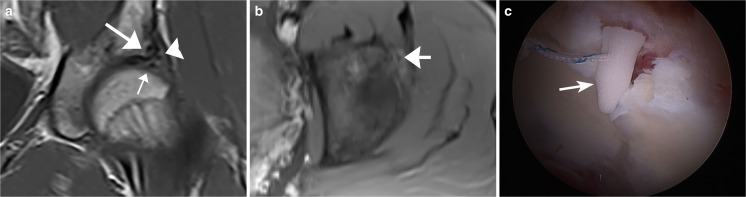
A 17-year-old boy with a catching and locking sensation 3 months after labral reattachment. a Coronal T1WI at 1.5 T shows a detached labrum (*small arrow*), the site of suture anchor placement (*large arrow*), and a nonspecific dark structure (*arrowhead*) that could represent a scar, but correlated with a displaced suture anchor at the time of arthroscopy. b Axial PD-weighted image at the same time as a shows the displaced anchor (*arrow*). The MRI findings were prospectively interpreted as possible displaced surgical suture material adjacent to the labral repair. c Arthroscopic image shortly after MRI shows that the suture anchor (*arrow*) has displaced from the acetabulum
